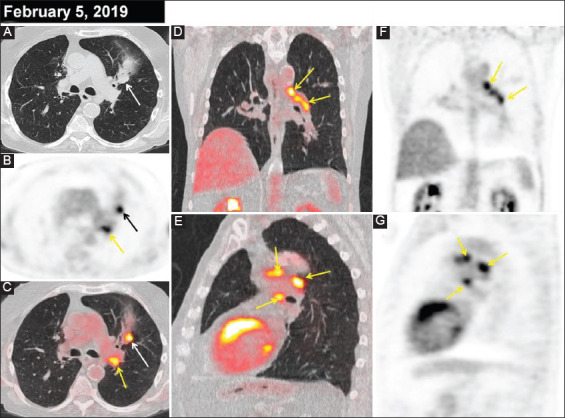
Same patient as in Figure 2. Tumor recurrence. 18F-Fludeoxyglucose (FDG) positron emission tomography/Computed tomography images in the axial (A, B, and C), coronal (D and E), and sagittal (F and G) plane show a nodule inside the scar, with FDG uptake and SUVmax 10 (white and black arrows). Left hilar and mediastinal lymphadenopathies have also appeared (yellow arrows).
